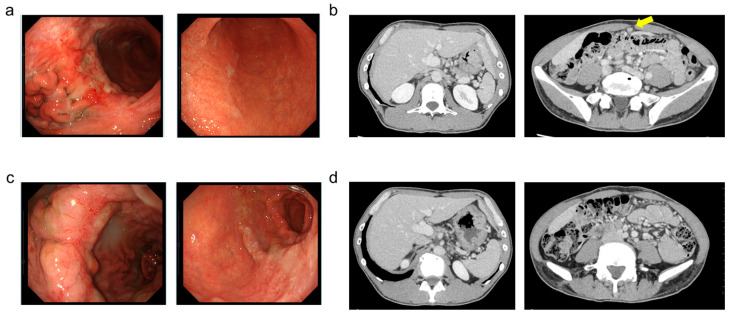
Radiological and endoscopic images. (a) Endoscopic images (before chemotherapy). (b) Computed tomography images (before chemotherapy)—arrow: peritoneal dissemination. (c) Endoscopic images (after chemotherapy). (d) Computed tomography images (after chemotherapy). (Improvement in gastric wall thickness from approximately 10–18 mm before treatment to 6–14 mm after chemotherapy).
